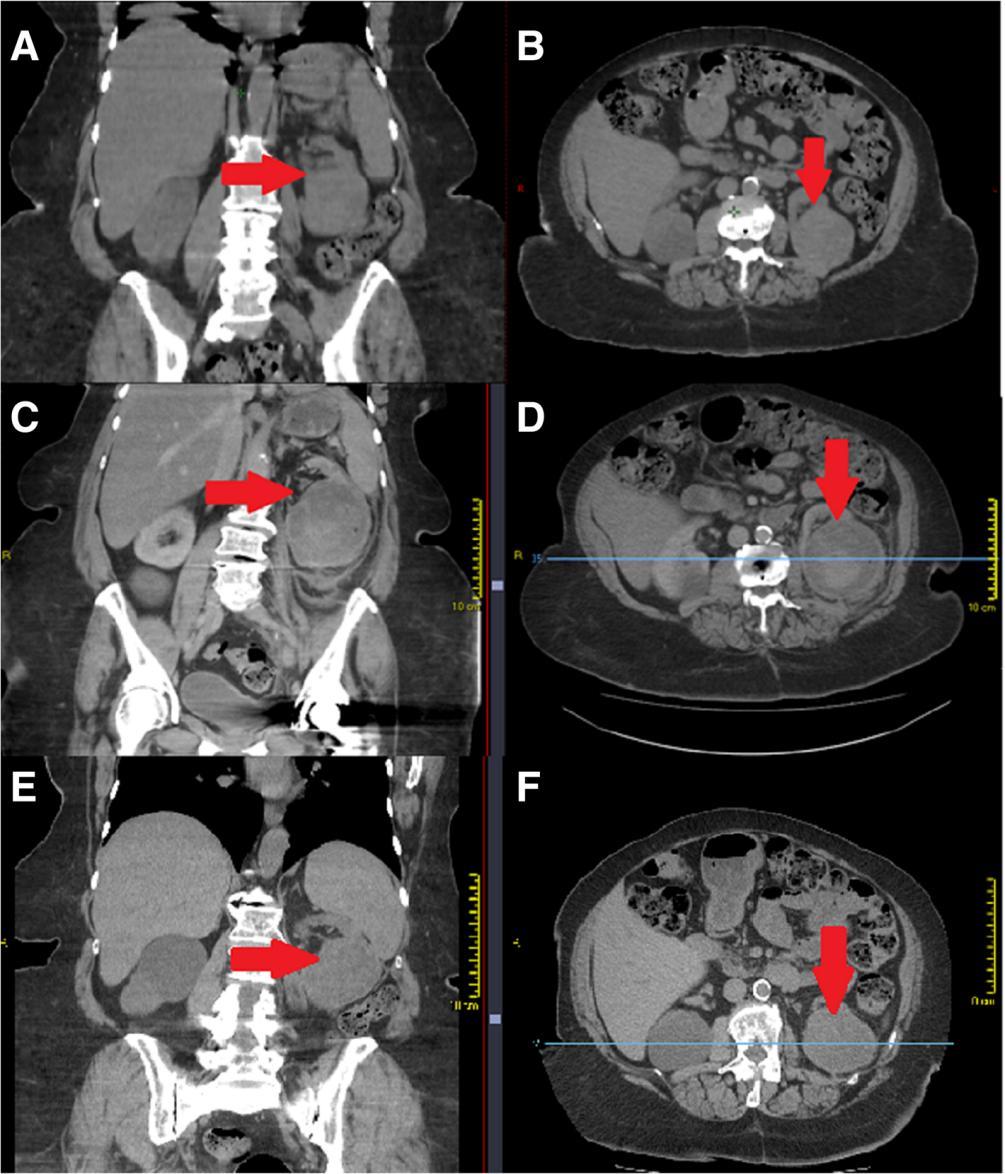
legend: RCC mass measuring 65 mm in its largest dimension at the time of planning CT (images a,b), compared to 87 mm mass with internal haemorrhage as seen in diagnostic CT after first of three prescribed ablative radiotherapy fractions (images c, d), and 60 mm at 18 months following adverse event (images e, f)
